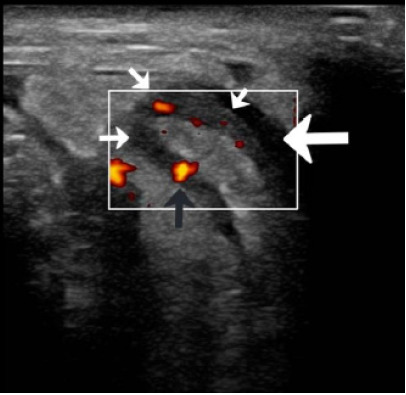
A blind-ended tubular structure, the edematous appendiceal wall (thin white arrows) with enhanced Doppler flow (black arrow) and free fluid (thick white arrow).
